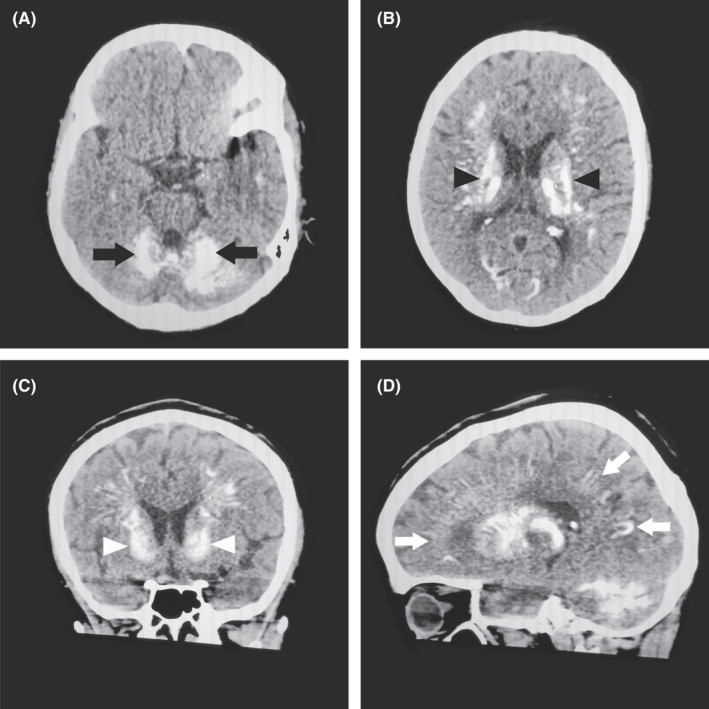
Plain computed tomography of the brain. Bilateral calcifications are seen in cerebellum (A), black arrows; basal ganglia (B) black arrowheads; thalami, periventricular region (C), white arrowheads; and subcortical fronto‐pareito‐occipital region (D), white arrows
